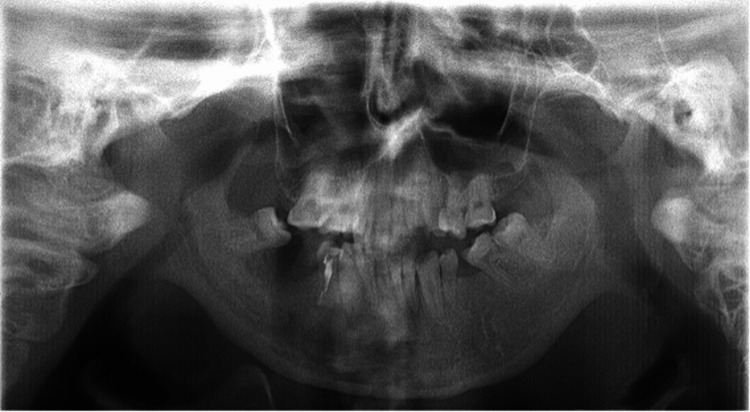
Panoramic radiograph. The patient was uncooperative, making it difficult to take the X-ray, so the image is from seven years ago
